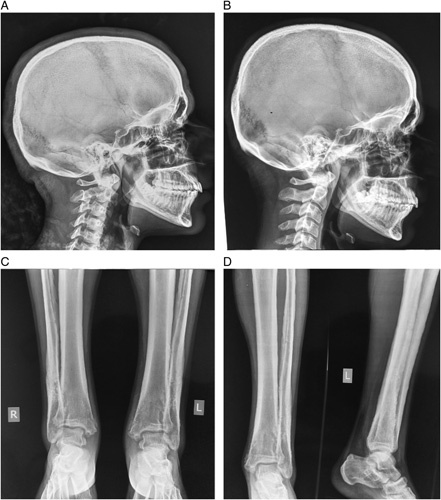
Radiograph showing (A) hyperostosis of skull bones and (B) Improvement in soft tissue thickening after etoricoxib treatment. (C and D) improvement in soft tissue swelling of bilateral ankle joint after 7 months of etoricoxib treatment.
